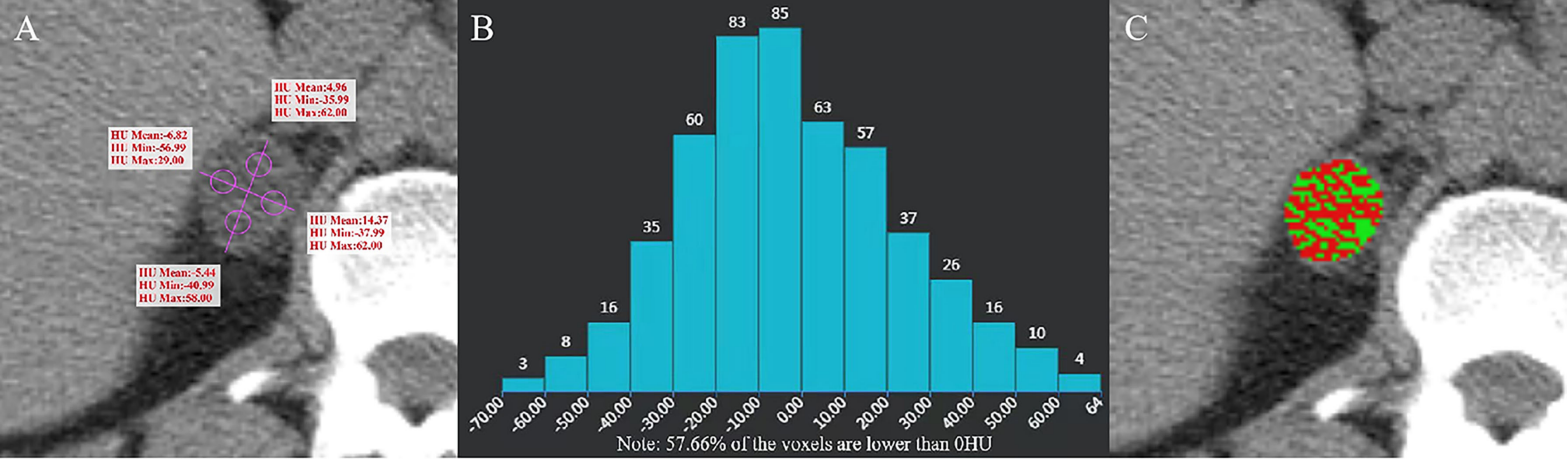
Adenoma of the right adrenal gland in a 47-year-old man. AVmean = 1.768 HU, AVmin = -42.99 HU (A), and CTH negative pixels = 57.66% (B). The CT value in the red area is ≤ 10 HU, and the CT value in the green area is > 10 HU (C).
